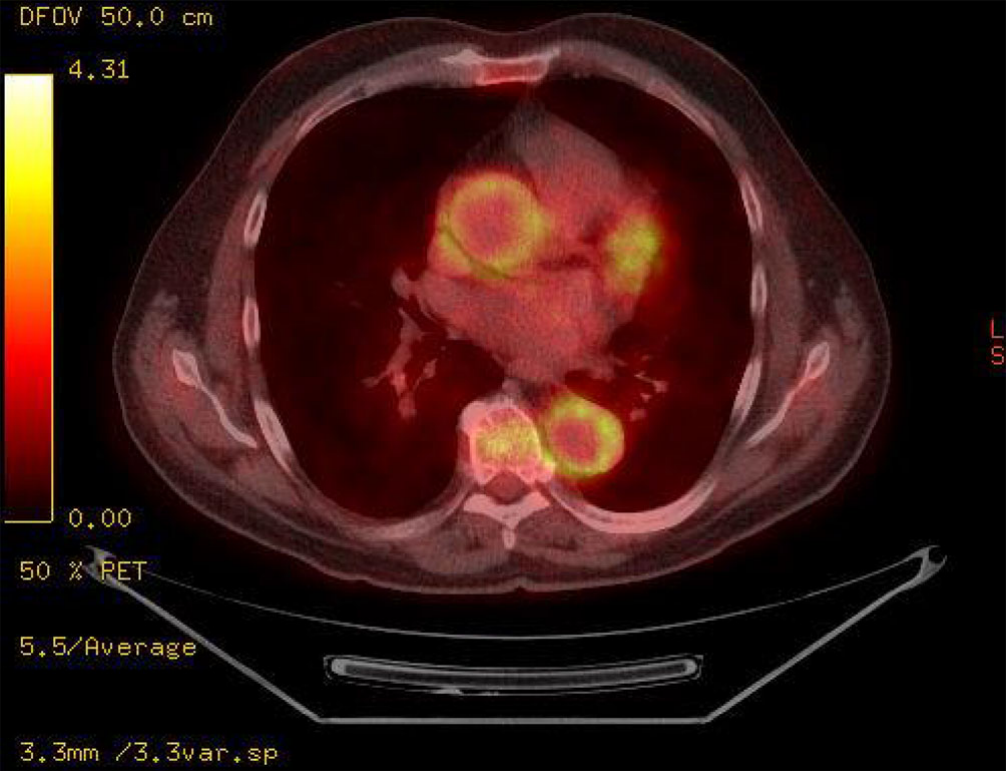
Female patient with Takayasu arteritis and positive positron emission tomography computed tomography showing marked enhancement around the aortic wall
